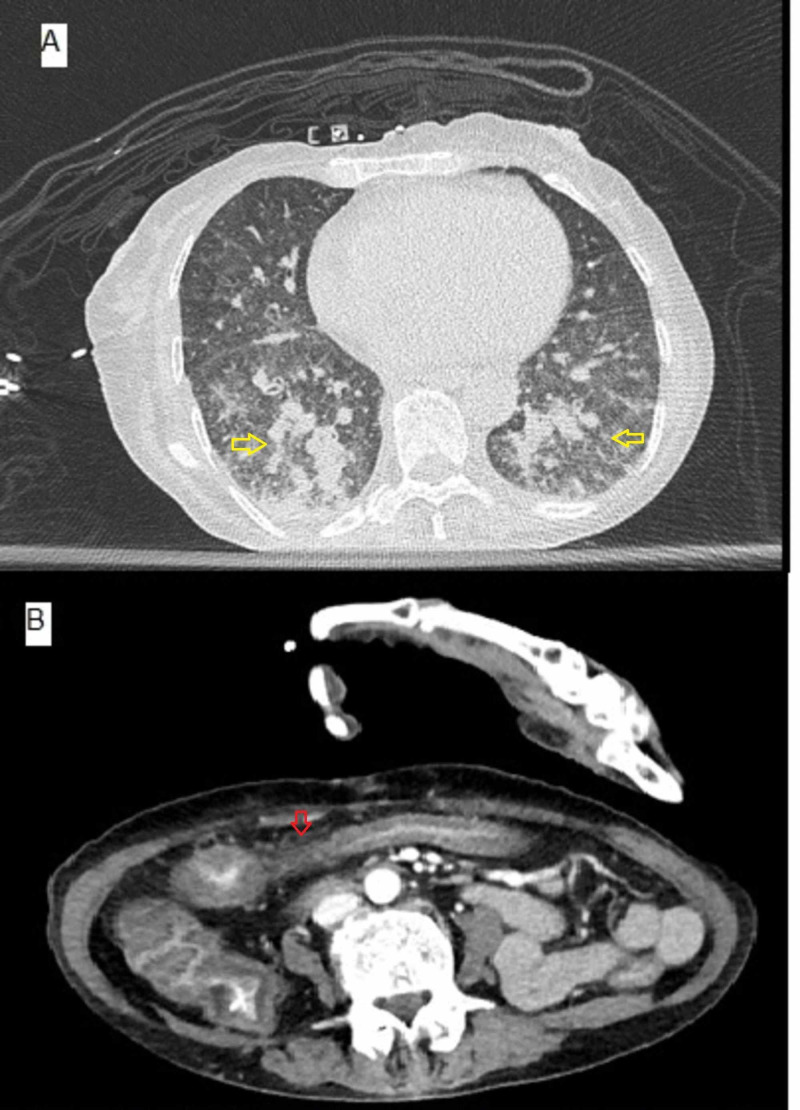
(A) CT chest with bilateral ground-glass opacities (yellow arrows). (B) CT abdomen with colitis (red arrow).
